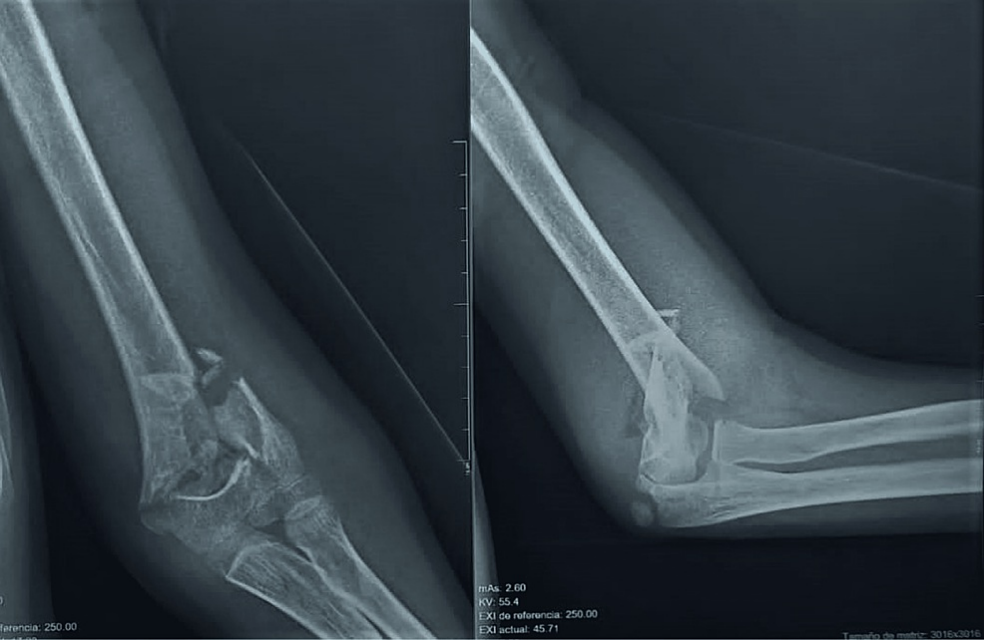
Radiographs showing left supracondylar humeral fracture, taken from an 11-year-old male patient with white hand sign and suspected compartment syndrome
